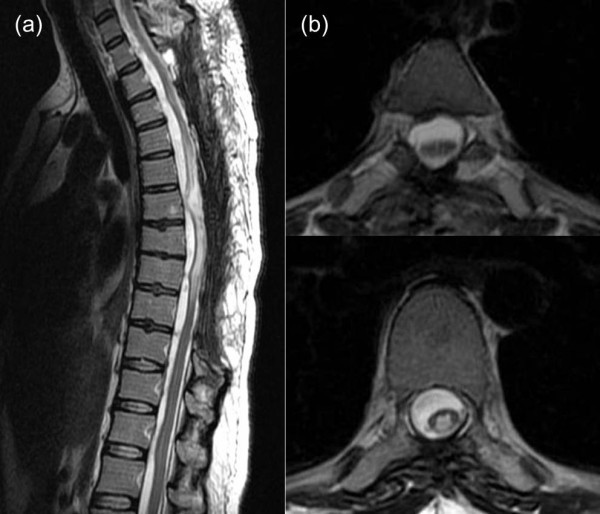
T2-weighted thoracolumbar MRI scan images obtained two months post-operatively. (a) Sagittal plane. (b) Coronal plane. The images show signal hyperintensity in the medulla and edematous changes in the spinal cord.
